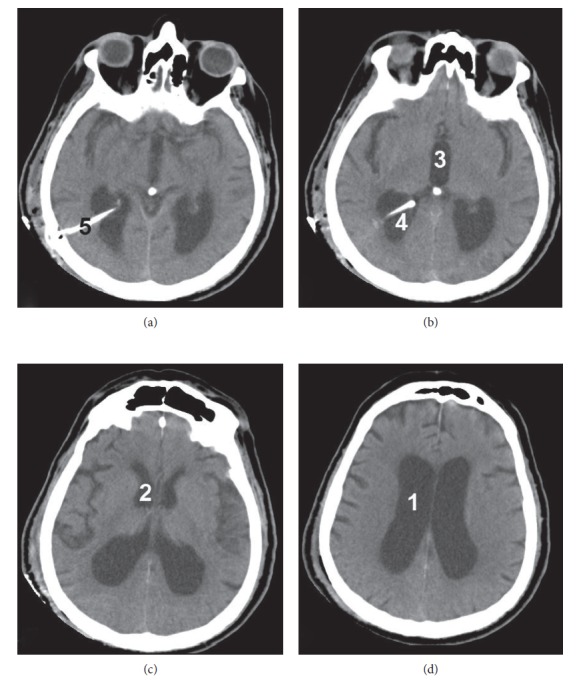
The cerebral CT scan of our patient. (a), (b), (c), (d) Different views (from inferior to superior). Note the large IVH within the right lateral ventricle (1), around the ventricular catheter (2), with extension into the third (3) and left lateral (4) ventricles. The right lateral ventricle is dilated (5), with apparent periventricular edema (6) and midline shift (7).
